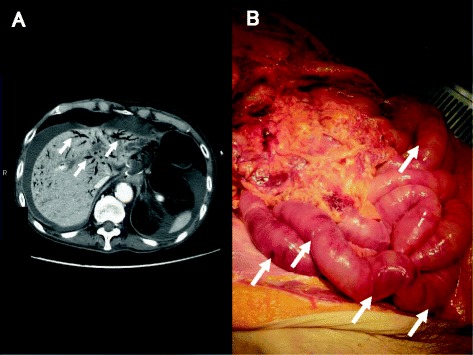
a CT for case 1. PVG spreads through the whole liver (*arrow*). b Autopsy photos for case 1. Segmental ischemic change was recognized at the wide range of the small intestine (*arrow*)
